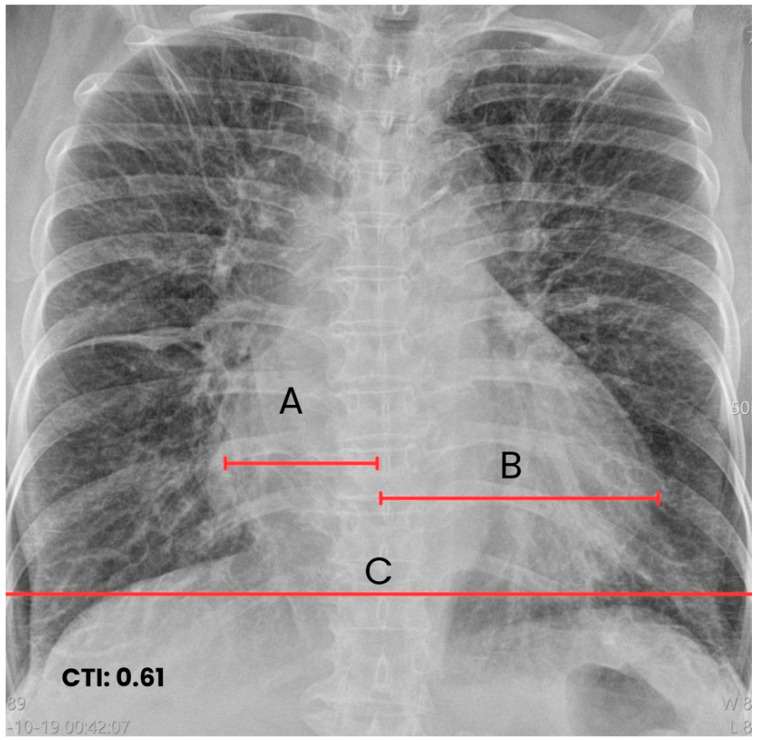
Posteroanterior chest radiograph. The image shows mild cardiomegaly with a cardiothoracic ratio of 0.61 (A + B/C), compatible with grade III cardiomegaly (red lines).
